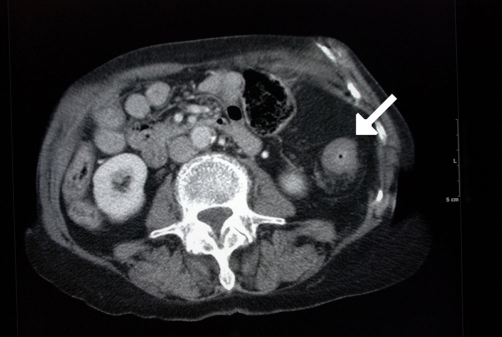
Abdominal CT showing an ischaemic stricture of the colon (arrow).
